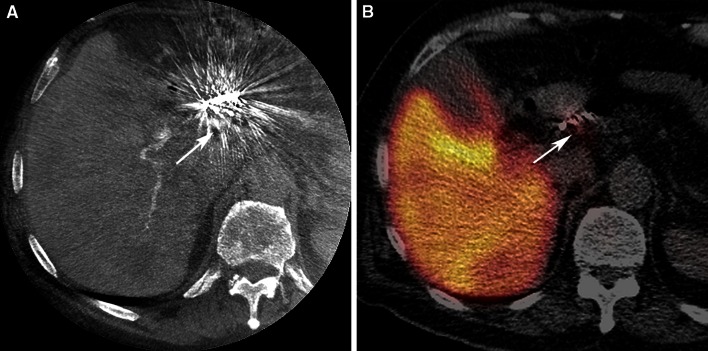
A Extrahepatic deposition of ^99m^Tc-MAA activity in the region of the coil embolized right gastric artery on a fusion SPECT/CT image (*white arrow*). B On C-arm CT imaging, no extrahepatic shunting was noted, due to the extensive coil-related beam hardening artifacts (*white arrow*)
